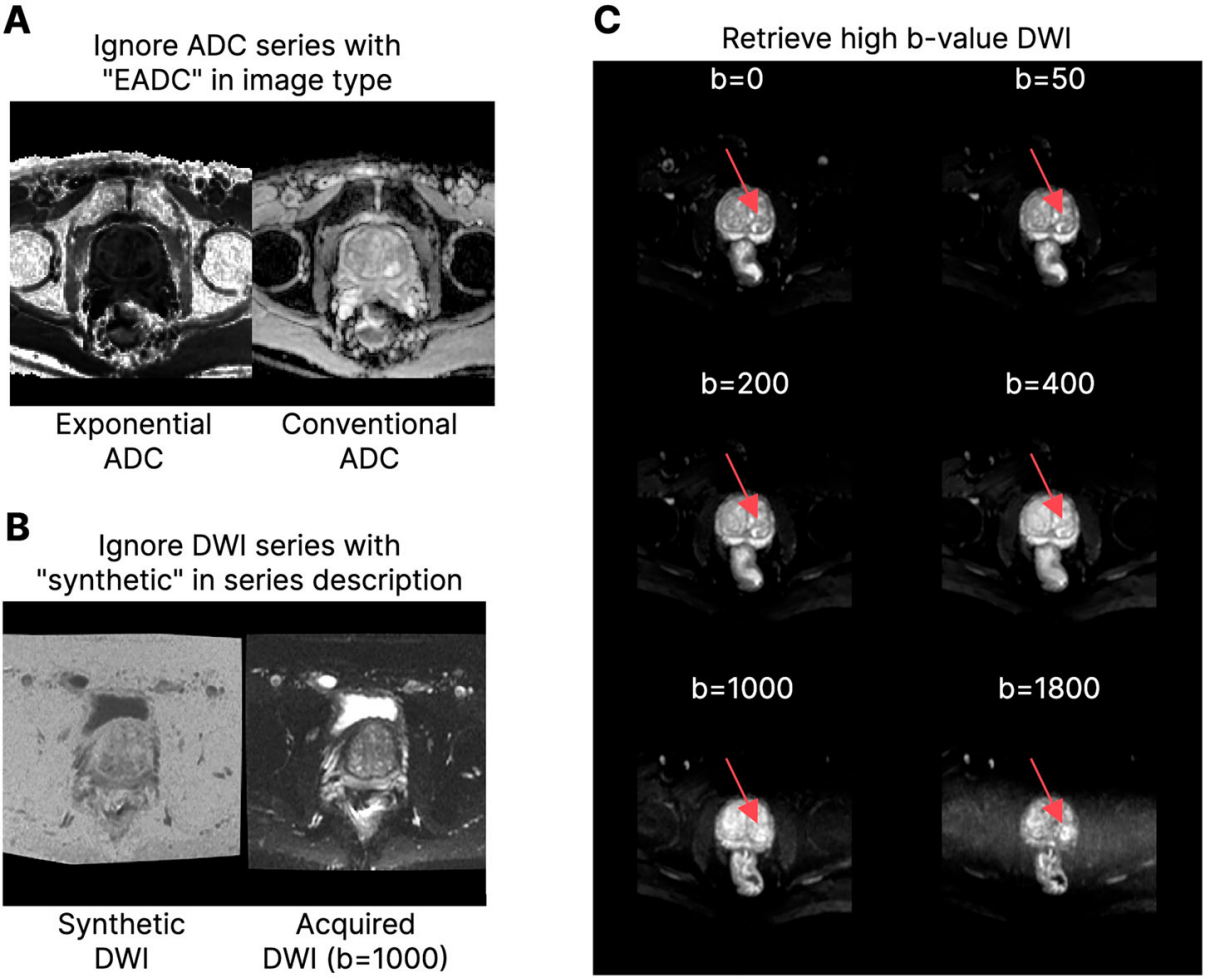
Visual examples of the ambiguities solved by heuristics. A Exclusion of exponential ADC (EADC) over conventional ADC. B Exclusion of synthetic DWI over acquired DWI. C Selection of a sufficiently high *b*-values allowing for better lesion detection (the red arrow represents the location of a potential lesion)
